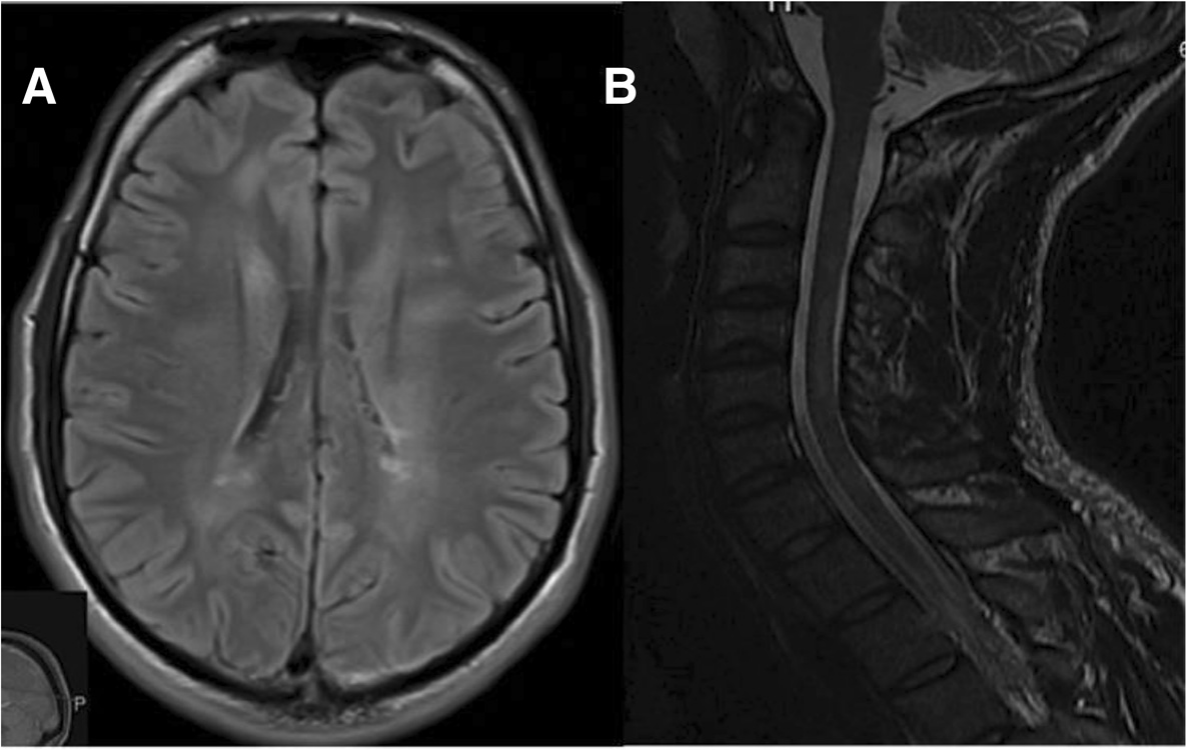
MRI brain and cervical spine images. A) Axial Fluid-Attenuated Inversion Recovery (FLAIR) image of the brain showing demyelinating plaques in periventricular and juxtacortical regions; B) Sagittal T2 image of the cervical spine showing intramedullary demyelinating plaques at C3 and C4-C5 levels.
